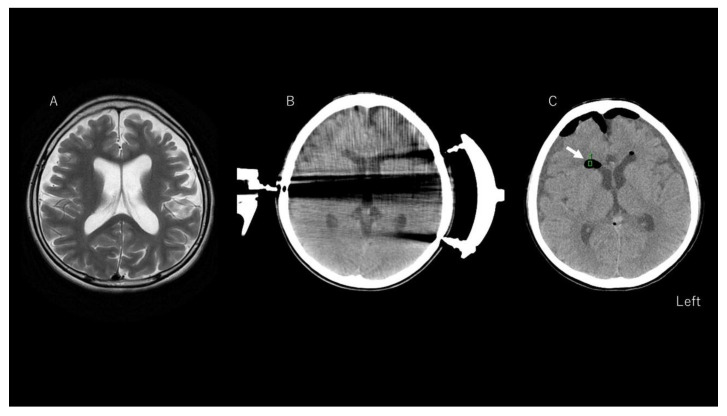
Results of neuroimaging in a representative case (Patient 56). T2-weighted imaging shows no structural lesions except for an atrophic appearance of the brain compared to norms for the patient’s age (A). A preoperative computed tomography (CT) image of the brain shows no intraventricular air density (B), even though halation artifacts from the Mayfield head holder are seen (this CT image was taken preoperatively for image guidance). A postoperative CT image (C) shows low density in the lateral ventricle (see arrow) representing pneumocephalus. The green square is the region of interest to calculate CT density (<−1000 HU).
